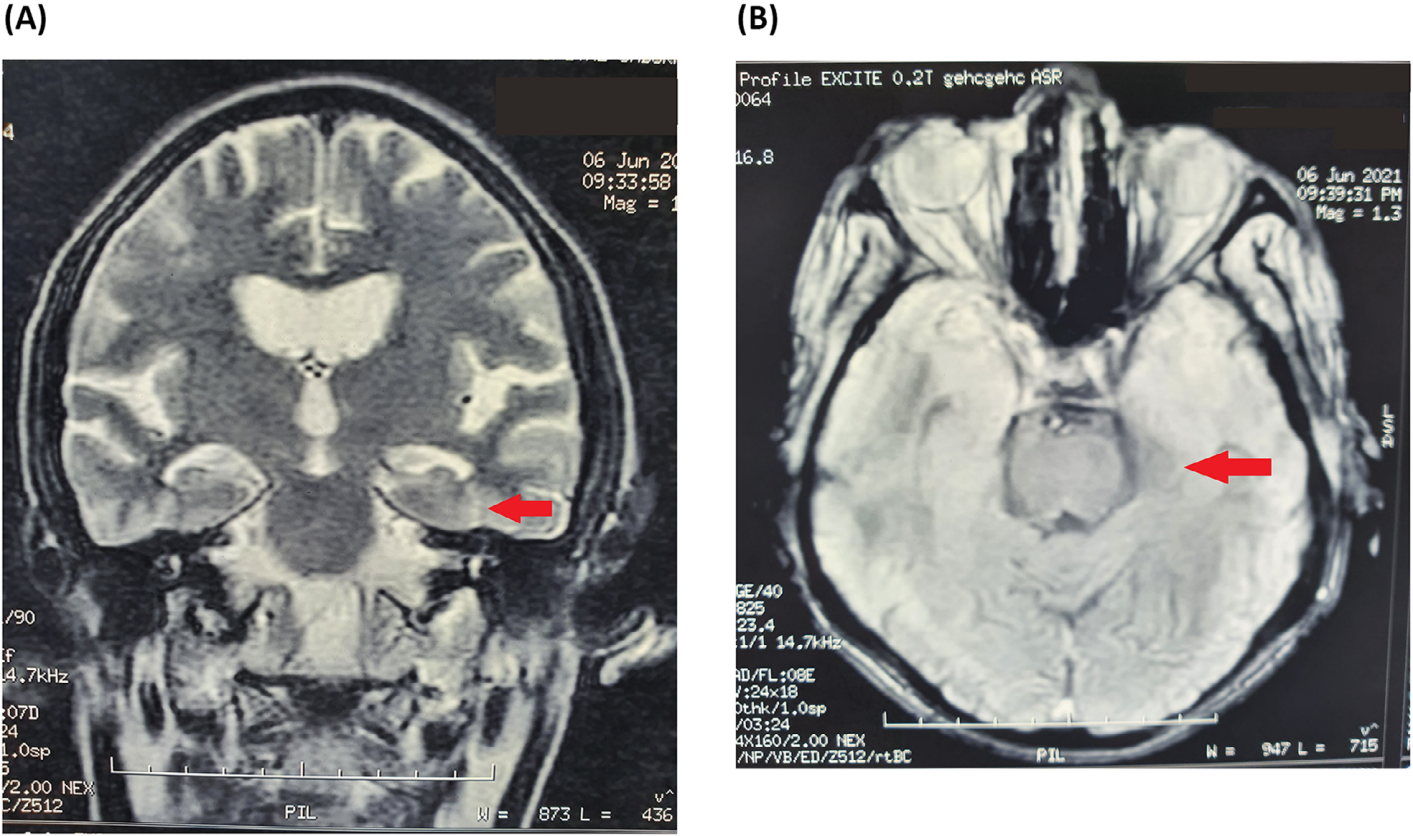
T2‐weighted MRI brain scans of patient 1 showing moderate hippocampal expansion on the left side, as indicated with the red arrow. (A) Coronal section. (B) Horizontal section.
